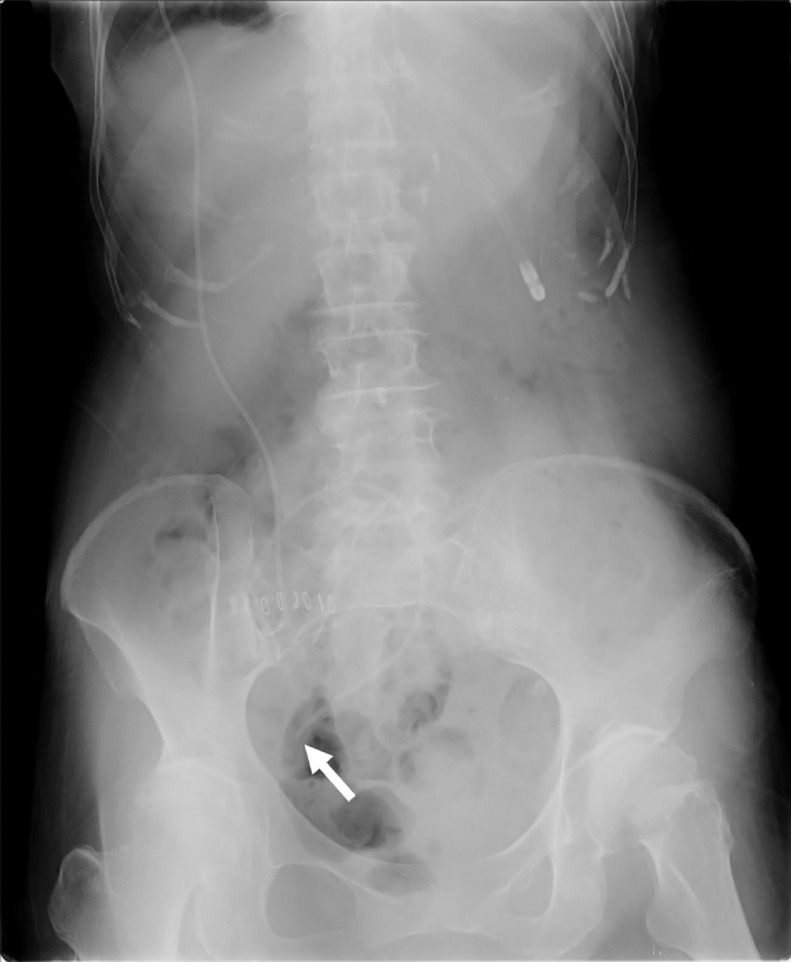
Abdominal radiograph taken right after the first ventriculoperitoneal shunt showing that the distal catheter is in the correct position. The white arrow indicates the tip of the catheter.
